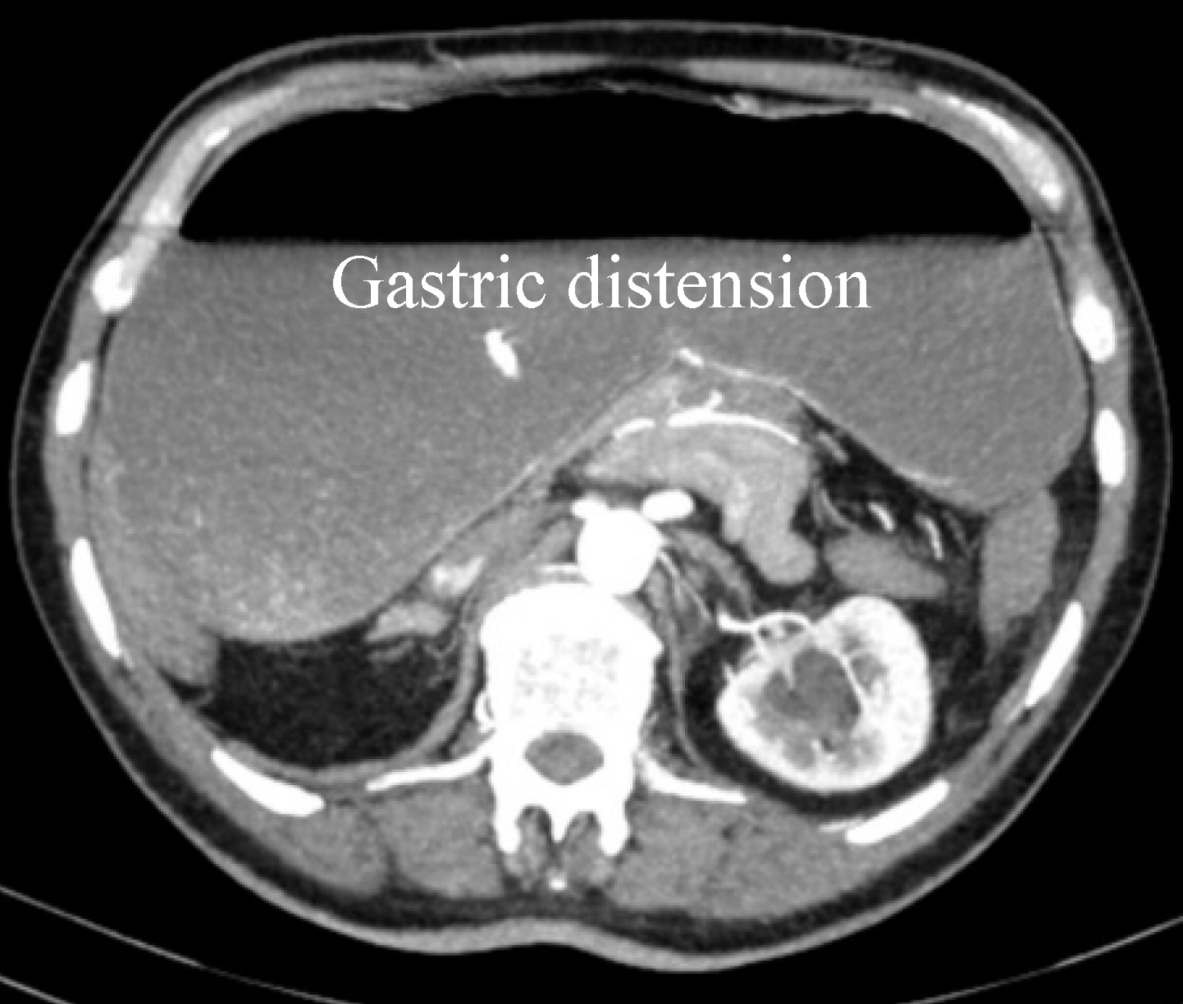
CT scan: massively distended stomach
